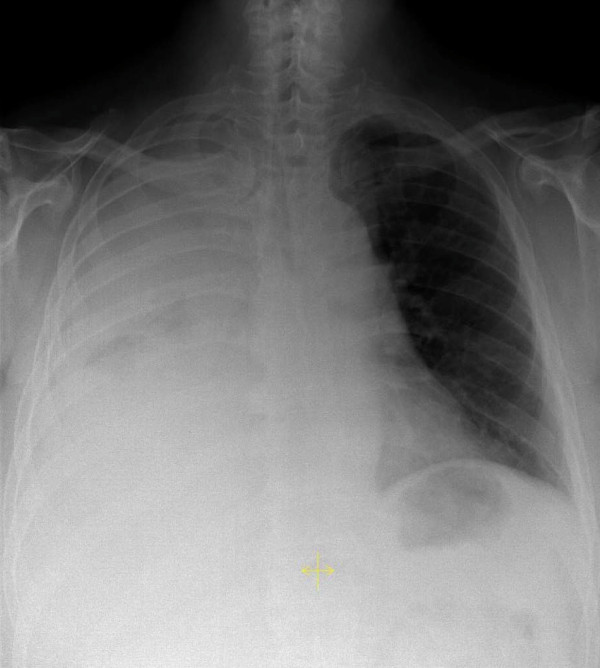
Initial posterior-anterior chest X-ray demonstrating a complete right-sided opacity, later diagnosed as chylothorax.
